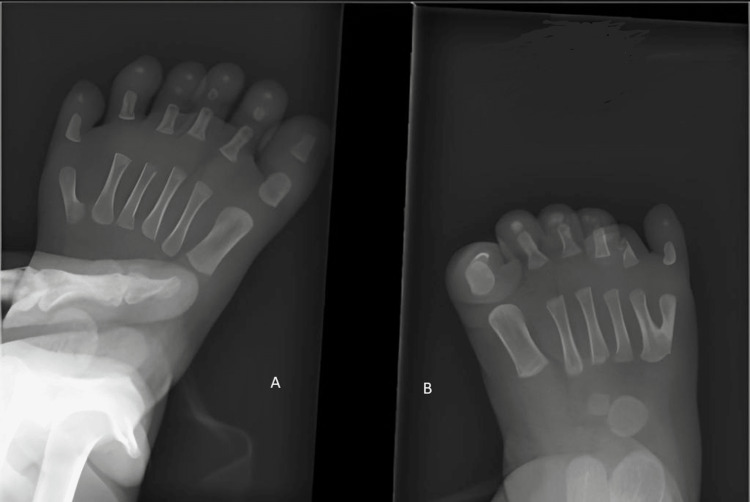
(A) X-ray of the left foot showing polydactyly. (B) X-ray of the right foot showing polydactyly.
